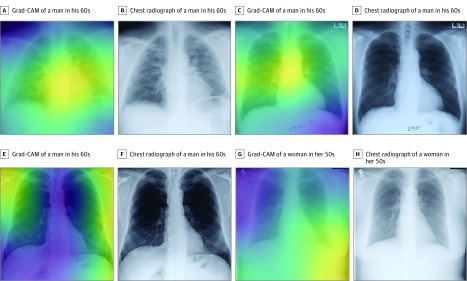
Gradient-Weighted Class Activation Maps (Grad-CAM) of Anatomy Contributing to the CXR-Risk Score A and B, Grad-CAM (A) and chest radiograph (B) of a man in his 60s from the Prostate, Lung, Colorectal, and Ovarian (PLCO) trial who died of respiratory illness in 2 years. Grad-CAM highlights an enlarged heart with prominent pulmonary vasculature indicating pulmonary edema (very high-risk CXR-risk score). C and D, Grad-CAM (C) and chest radiograph (D) of a man in his 60s in the PLCO trial who died of cardiovascular illness in 7 years. Grad-CAM highlights the mediastinum and aortic knob, which may indicate cardiovascular health; sternotomy wires indicate previous cardiothoracic surgery (very high-risk CXR-risk score). E and F, Grad-CAM (E) and chest radiograph (F) of a man in his 60s in the National Lung Screening Trial who was alive at the end of 6-years follow-up. Grad-CAM highlights the extrathoracic soft-tissues, which may reflect body habitus (low-risk CXR-risk score). G and H, Grad-CAM (G) and chest radiograph (H) of a woman in her 50s in the PLCO trial who was alive at the end of 9-years follow-up. Grad-CAM highlights the shadow of the left breast and waist, which convey information about sex and habitus, important determinants of longevity (very low-risk CXR-risk score).
